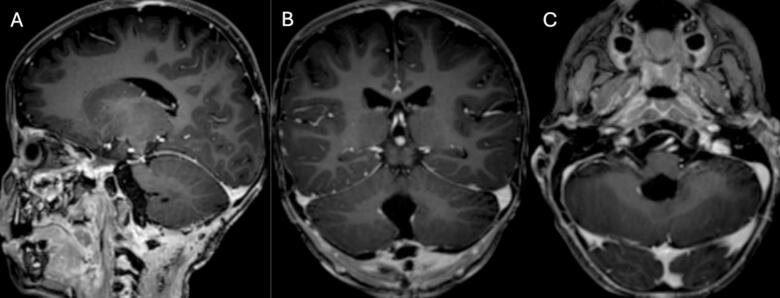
Magnetic resonance imaging of the brain: Second follow-up imaging showing total remission.
